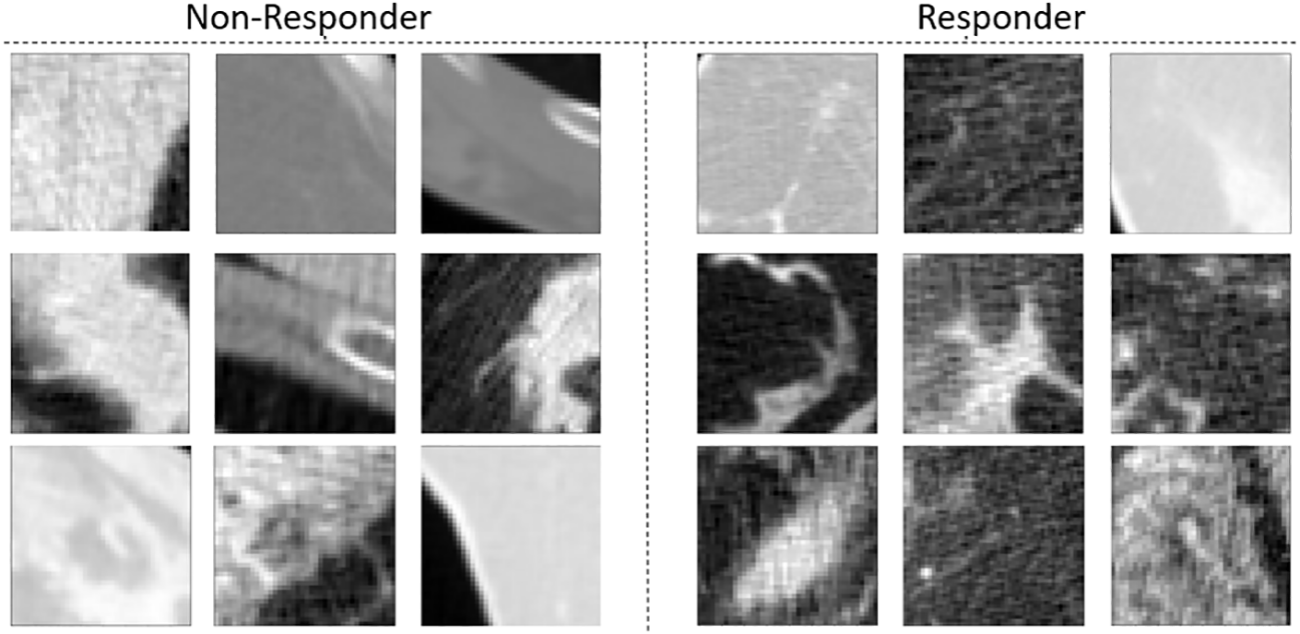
CT images of tumors of patients with LABC who did not respond to treatment (left) and tumors of patients with LABC who did respond to treatment (right).
